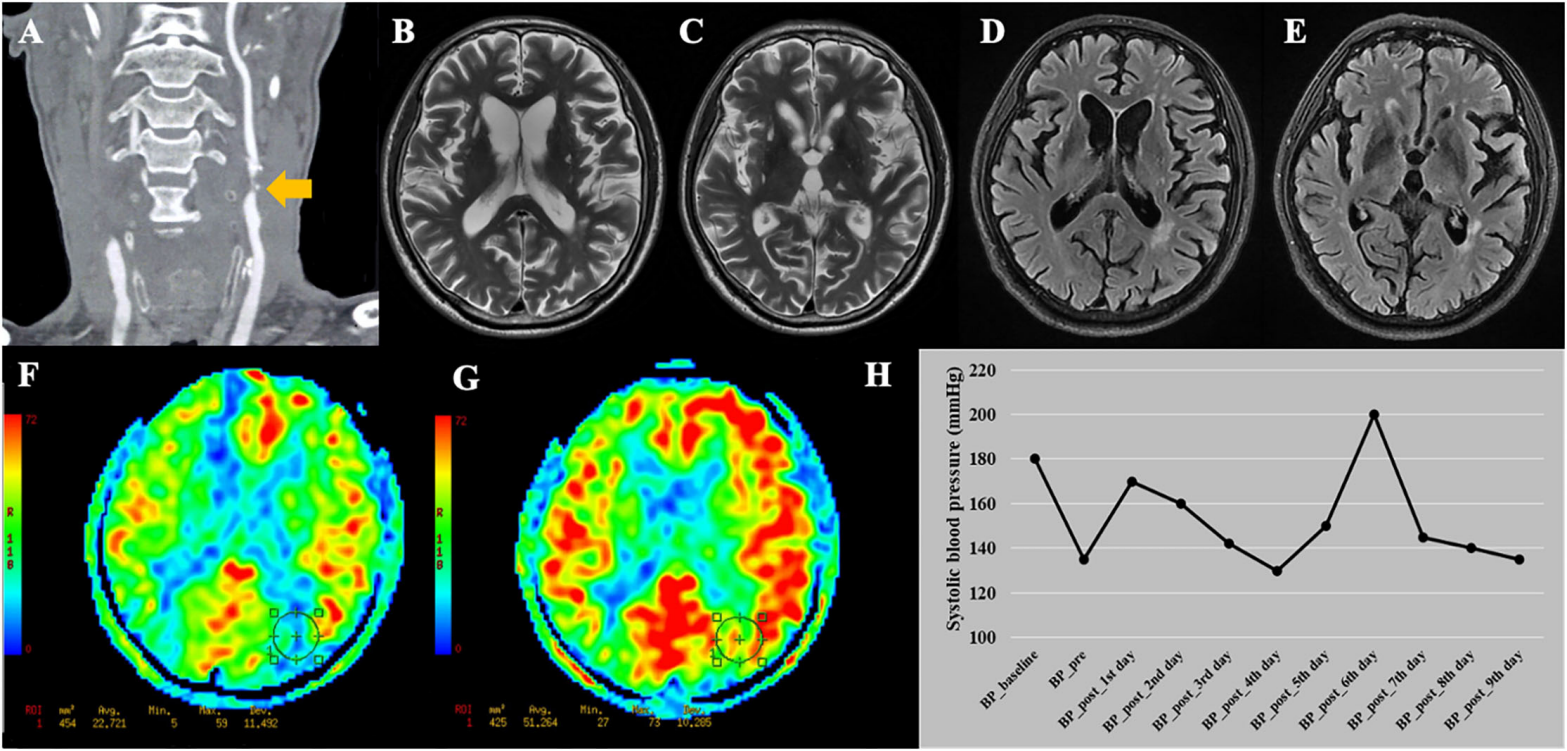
A representative case of cerebral hyperperfusion syndrome. (A) This patient was diagnosed with bilateral severe carotid stenosis. During this hospitalization, this patient received carotid endarterectomy (CEA) on the left side. (B–E) Pre-operative T2WI and FLAIR show Fazekas score of 4 points and multiple lacunes in bilateral basal ganglia and left thalamus. (F) Pre-operative arterial spin labeling image shows a significantly decreased cerebral blood flow (22.72 ml/100 g/min) in the watershed area. (G) Post-operative arterial spin labeling image shows the cerebral blood flow (51.26 ml/100 g/min) increase >100% compared with pre-operative values. (H) This patient developed cerebral hyperperfusion syndrome during the first day after CEA with systolic blood pressure of 170 mmHg. The patient experienced dysphoria, delirium under fluctuating blood pressure. After adjusting the medication, the patient discharged with stable blood pressure. BP_baseline, baseline systolic blood pressure on admission; BP_pre, systolic blood pressure measured the day before surgery; BP_post, systolic blood pressure measured everyday after CEA before discharge.
